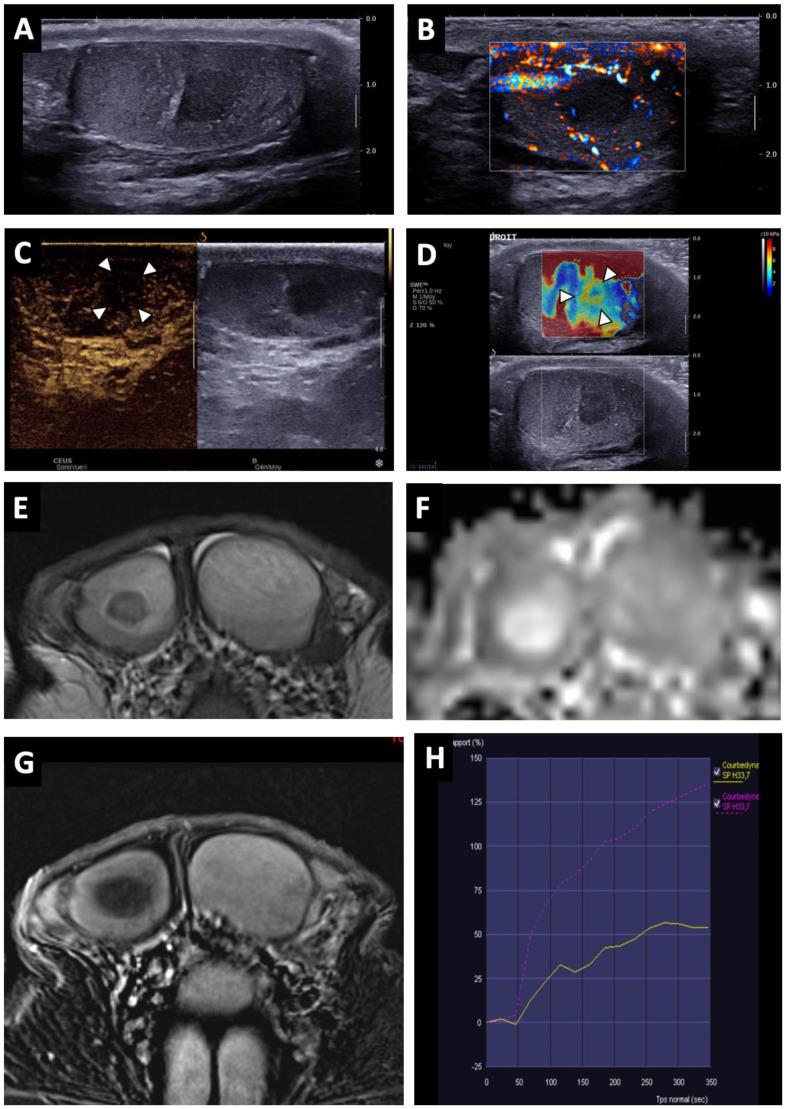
BOTT of the right testis in a 41-year-old man (infertility screening). Transverse B-mode US showed an ill-delineated hypoechoic nodular area (A). With colour Doppler US, the hypoechoic nodule was frankly hypovascular upon Ultrasensitive Doppler (B). CEUS demonstrated a hypo-enhanced (white arrowheads) area in concordance with the B-mode nodule (note that even the adjacent testis parenchyma was also poorly enhanced) (C). SWE map showed an area of increased stiffness in concordance with B-mode nodule (D). MRI axial T2W image showing a nodule with a hypo-intense signal (white arrow) (E). ADC map of the diffusion sequence demonstrated a focal area with an elevated ADC value compared with the contralateral testis (F). After the intravenous injection of gadolinium chelate, an area of reduced enhancement of the nodule was visible (G). Nodule enhancement followed the yellow time–signal-intensity curve (type 1) and the contralateral parenchyma corresponded to the purple one (H).
